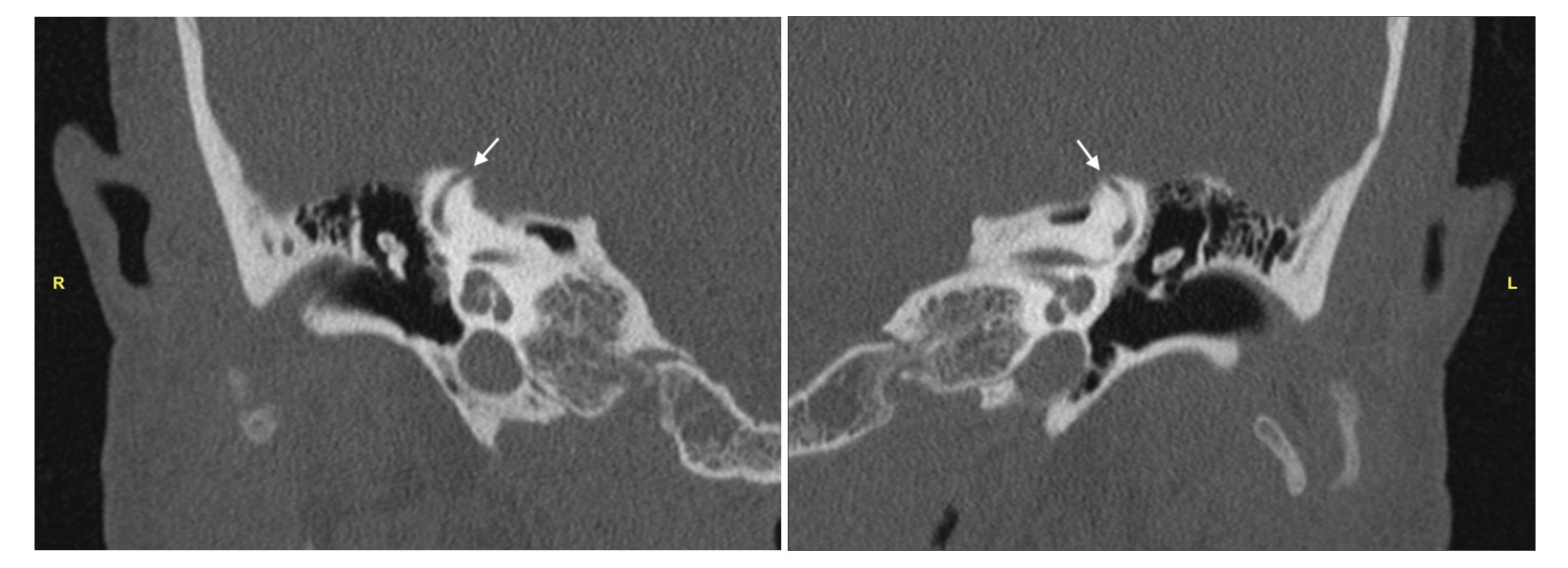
Computed Tomography of Temporal Bones High-resolution computed tomography scans of the temporal bones demonstrating the area of dehiscence (arrow) at the apex of the (R) right superior semicircular canal and (L) left superior semicircular canal
